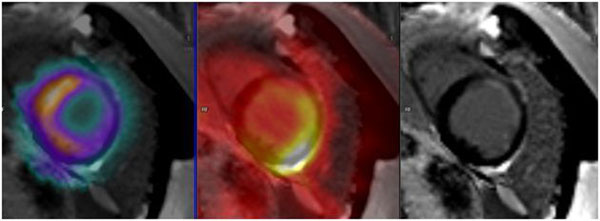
(from right to left: Tc-99-SPECT, F-18-FDG-PET, PSIR-Late Enhancement) While CMR shows subendocardial scar in the anterior and lateral segments, PET shows an intense glucose metabolism in the lateral wall. However the inferior and inferoseptal segments show low glucose metabolism although viable in MRI. An additionally performed TC-99-SPECT could exclude a perfusion deficit at rest, consistent with viable myocardium in the late enhancement sequence.
